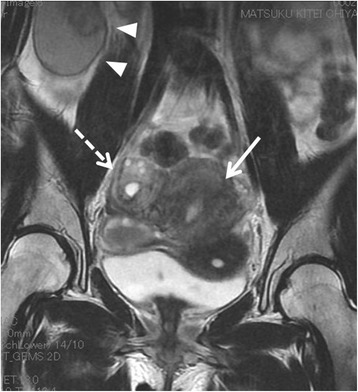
MRI demonstrates a cystic mass of iso-intensity on T1-weighted and high intensity on T2-weighted images (arrow heads), uterine fibroids (arrow), and cystic masses at the right ovary (broken arrow)
